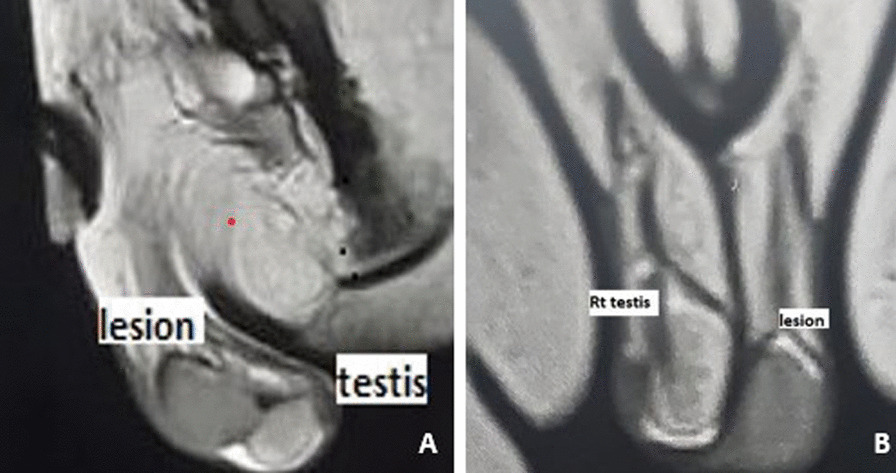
Magnetic resonance imaging. A Sagittal and (B) coronal T2 sequence revealed well defined extratesticular hypointense mass lesion
